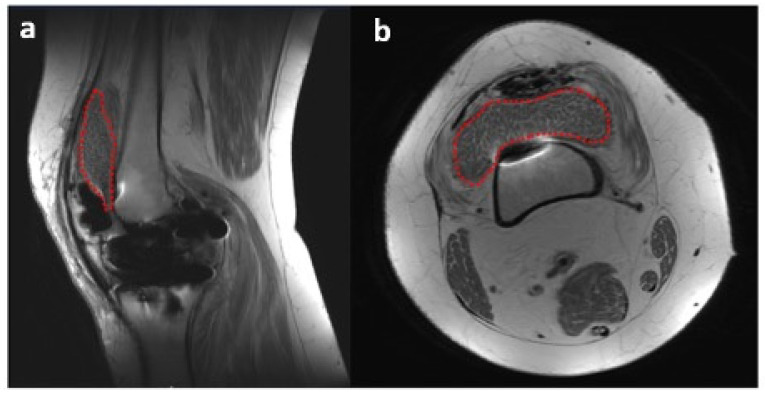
MRI of right knee 3 October 2022 (GE, Coil: HD TRknee PA): (a)—sagittal plane (proton density—PD), (b)—axial plane (PD). Dotted red line outlines the appearance of diffuse rice bodies in the anterior compartment and medial and lateral gutters.
